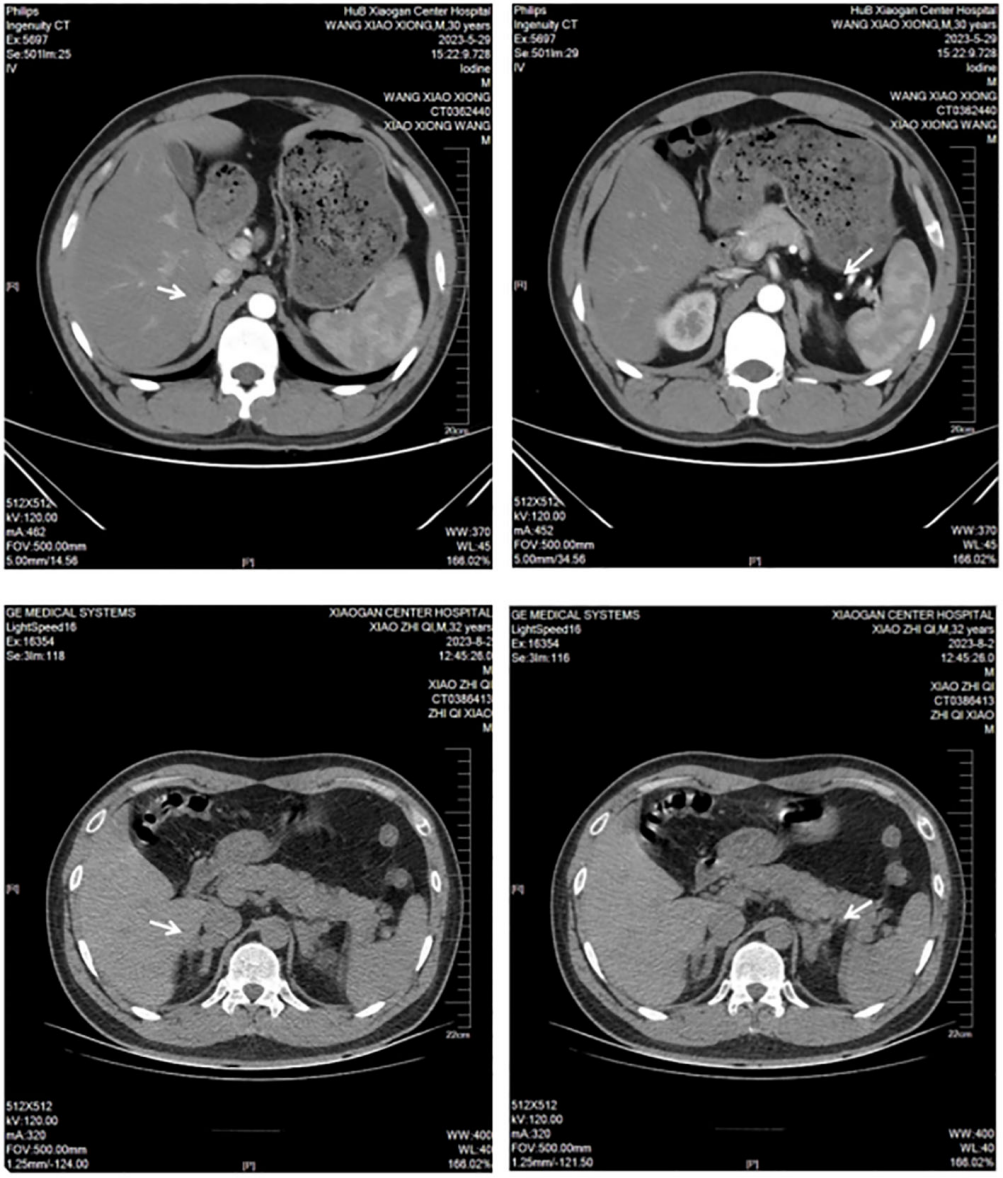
Adrenal CT images for Case 1 and Case 2.
